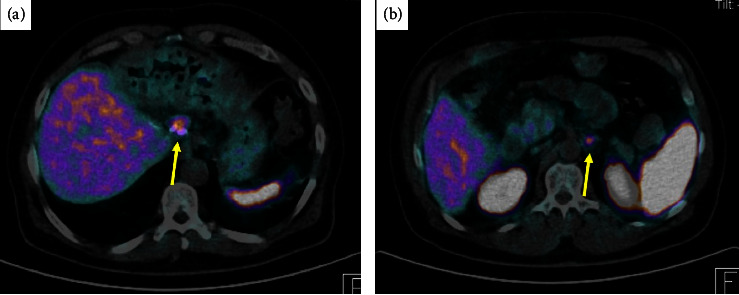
Gallium-DOTATATE whole body transversal view showing (a) right para gastric lymph node 2.6 × 1.4 cm SUV max 8.9 (yellow arrow) and (b) left para-aortic lymph node 1.2 × 0.9 cm SUV max 7.2 (yellow arrow).
